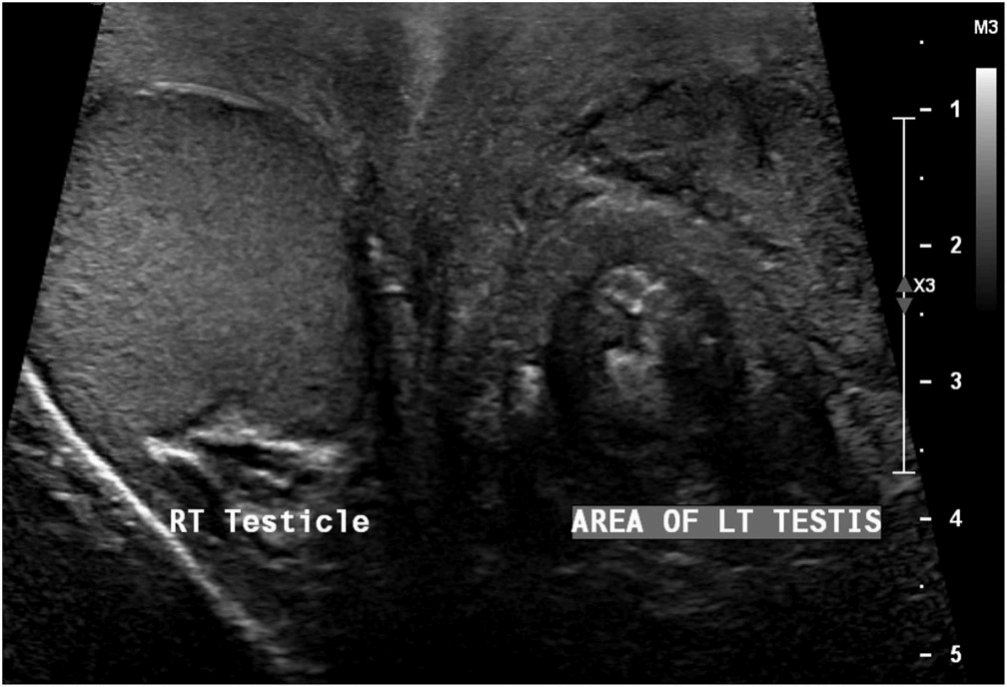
Ultrasound showing an atrophic left testis with heterogenous hyperechoic and hypoechoic areas along with collection in the scrotal wall.
